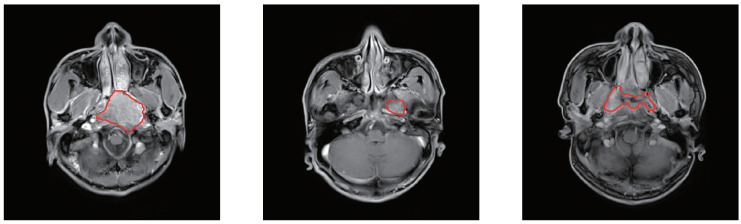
MRI slices of head and neck and tumour location of nasopharyngeal carcinoma. The area delineated by the red line is the result of the doctor’s manual segmentation.
